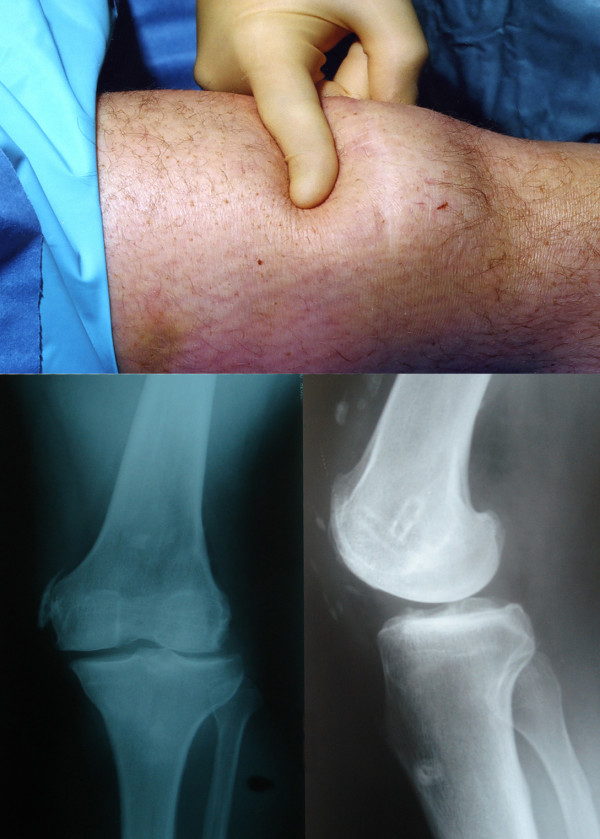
Clinical picture showing positive "sulcus sign" in the suprapatellar region, and the radiographs showing heterotopic ossification, absence of patella, and soft tissue shadow defect anterior to the distal femur.
